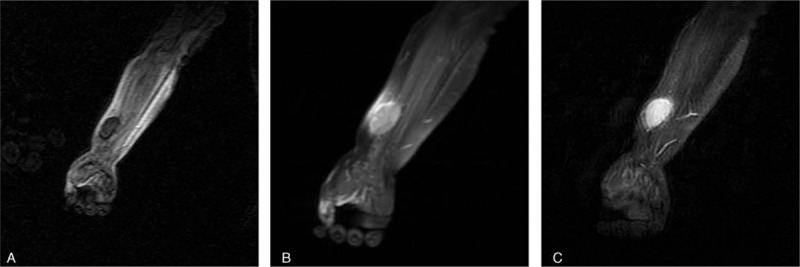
MRI of STRDD. (A) Note that lesion has a low signal intensity on T1-weighted image. (B) High signal intensity on T2-weighted MRI. (C) After injection of gadolinium contrast agent, a strong enhancement can be noted.
